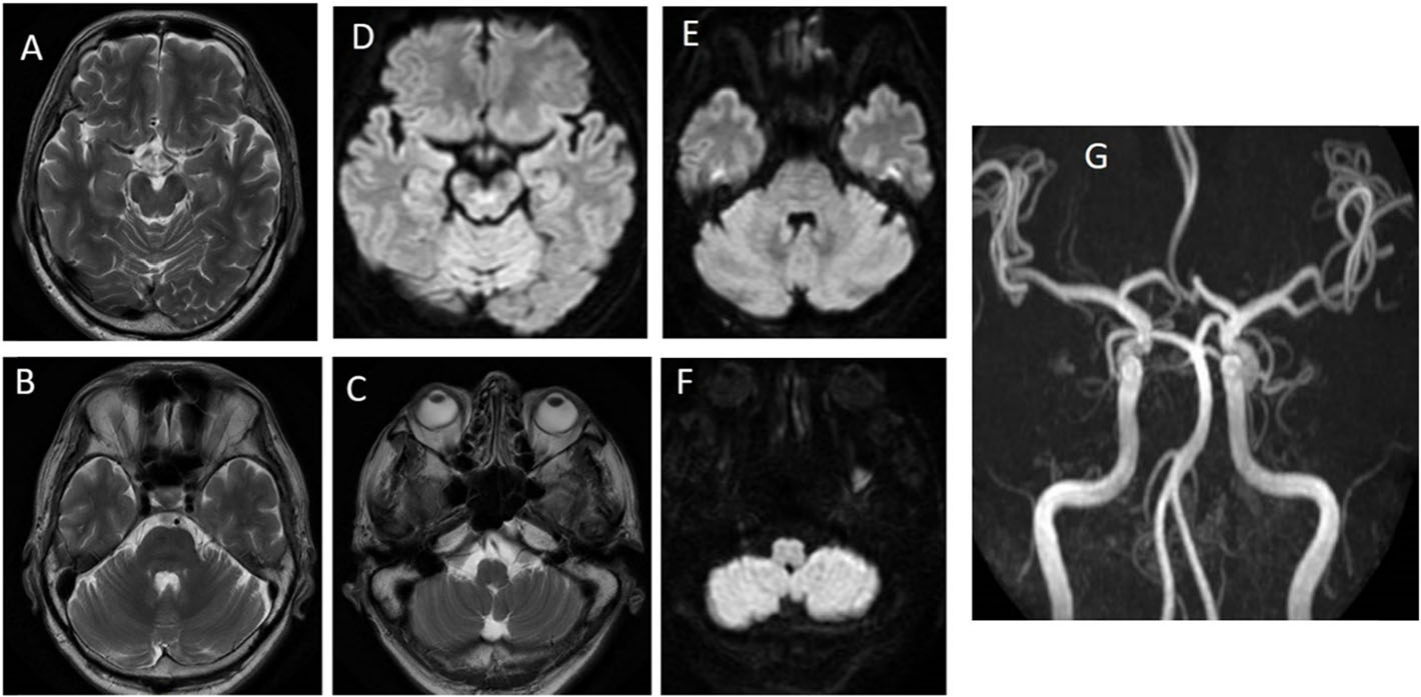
Magnetic resonance imaging of the brain showing T2 weighted images of (a) midbrain (b) pons (c) medulla and diffusion weighted images of (d) midbrain (e) pons (f) medulla. (g) Magnetic resonance angiogram
